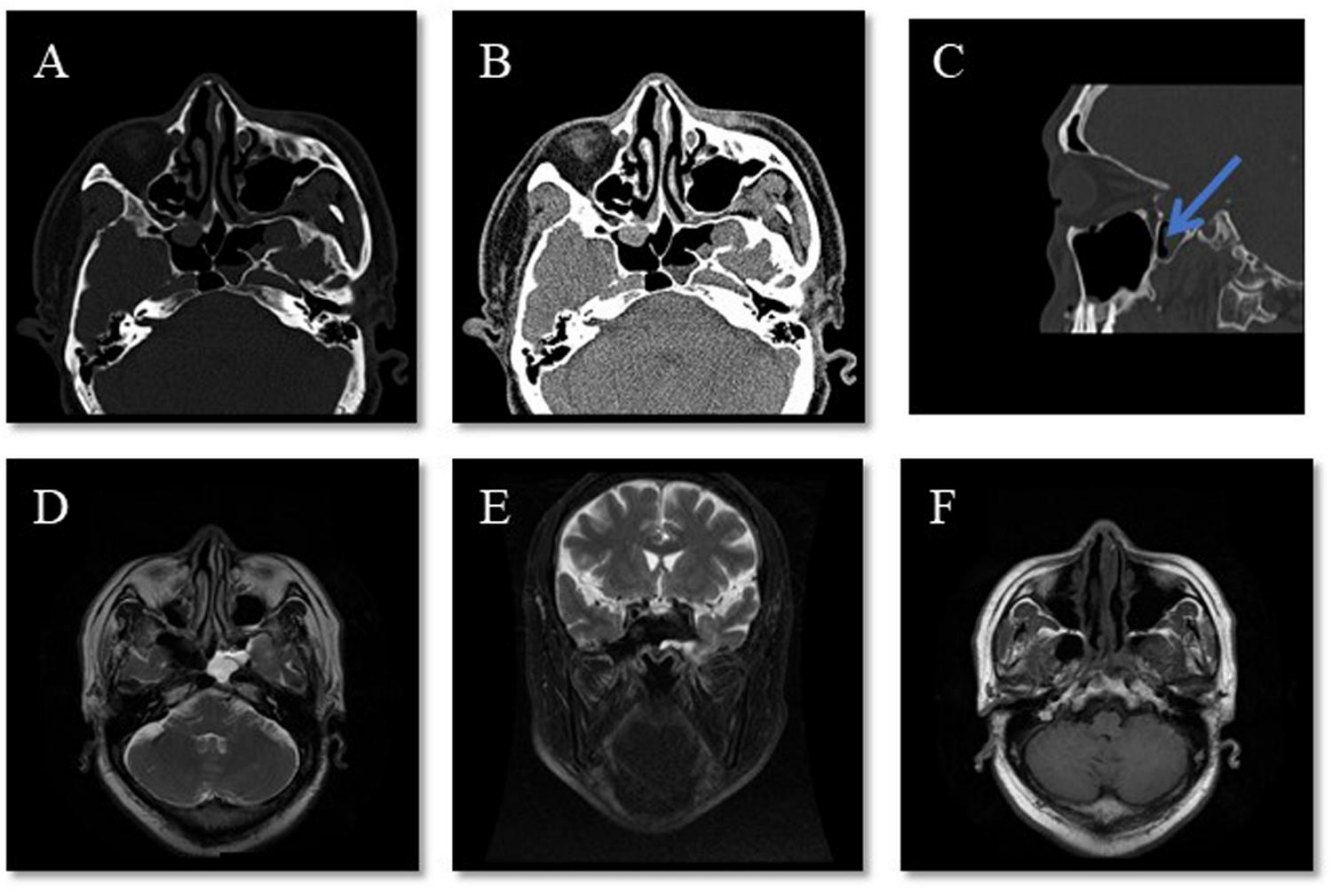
Representative radiographical images upon admission. (A–C) CT of the patient’s sinuses shows a continuity disruption in the lateral wall of the left sphenoid sinus and meningoencephalocele in the sinuses. The arrow indicates direct apposition between the sphenoid sinus recess and the posterior maxillary wall without intervening vascular structures. (D–F) MRI of the sinuses and brain shows effusion in the left sphenoid sinus and an abnormal cystic signal, which raised suspicion of it being brain tissue.
